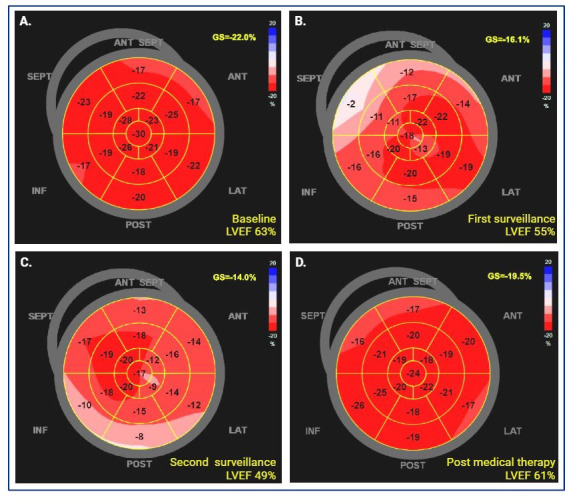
Global longitudinal strain (GLS) images of a 63-year-old female with Grade 1 invasive ductal carcinoma of the breast, estrogen receptor (ER)/progesterone receptor (PR)/human epidermal growth factor receptor-2 (HER2) receptor positive. She was treated with lumpectomy and adjuvant trastuzumab. (A) Baseline transthoracic echocardiogram (TTE) with normal GLS and left ventricular ejection fraction (LVEF), prior to receiving trastuzumab. (B) First surveillance TTE after commencement of trastuzumab therapy showed a decline in LVEF of 8% (less than the 10% cutoff for cardiotoxicity), an absolute decline in GLS of 6%, and a relative decline in GLS of 27%, consistent with mild CTRCD. (C) Second surveillance TTE after commencement of trastuzumab showing further reduction in LVEF to 49% and GLS to 14%. Patient was accordingly commenced on guideline-directed medical therapy (GDMT), including beta-blocker and angiotensin receptor blocker, and advised for cessation of alcohol (which she was taking in excess). No interruption in trastuzumab therapy was needed, as LVEF did not drop further with these measures. (D) TTE 6 months after GDMT and on completion of trastuzumab therapy with improvement in both LVEF and GLS.
